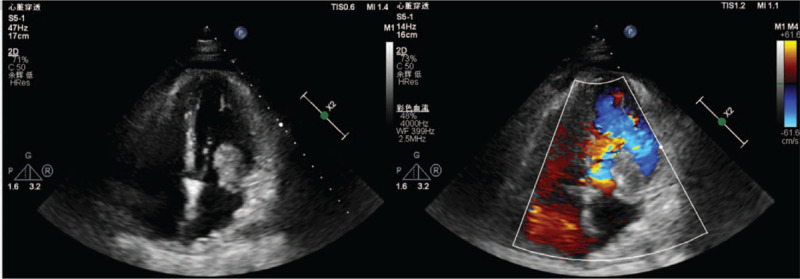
Echocardiography was performed 3 months after surgery and the tumor recurred in the posterior lobe of the mitral valve.
